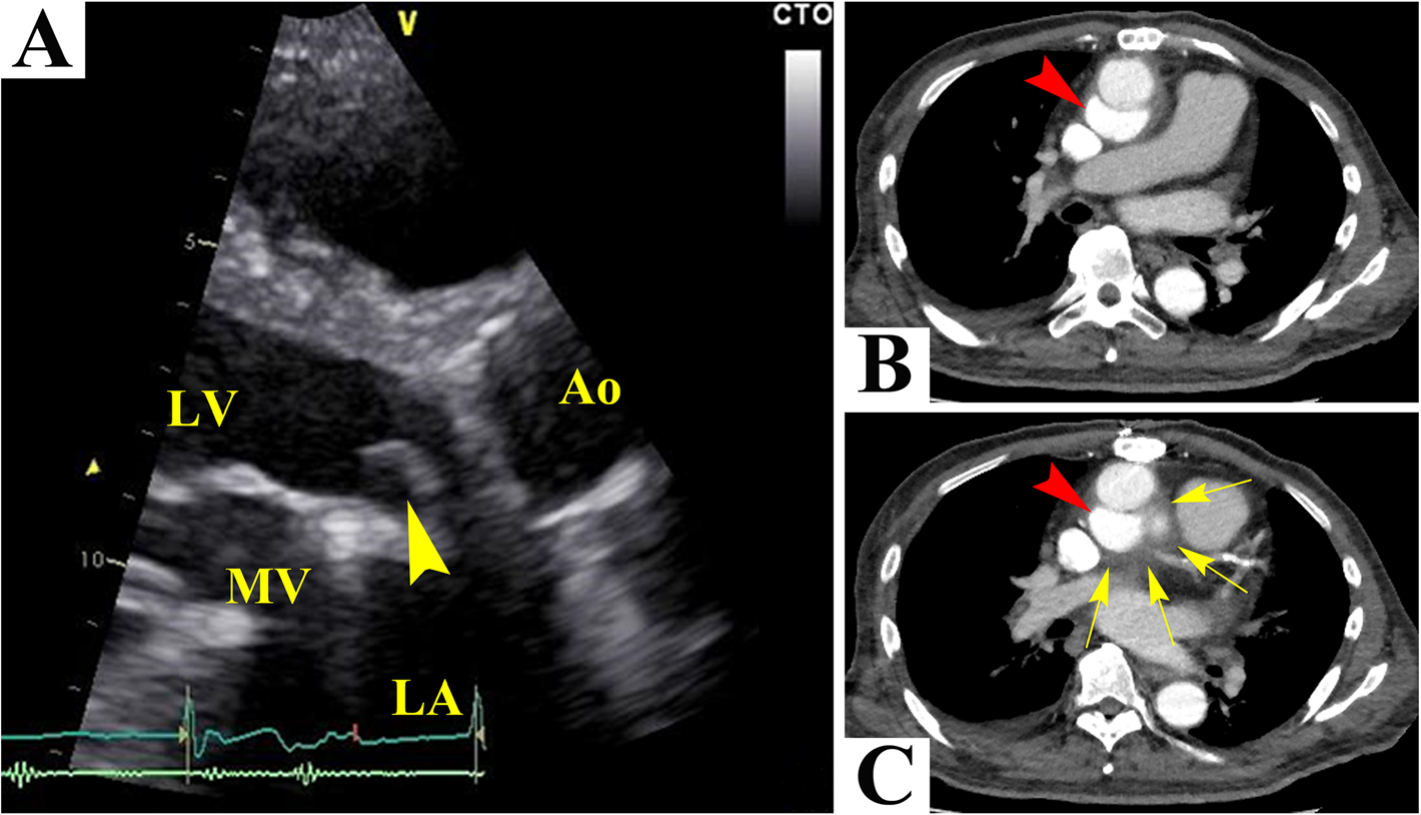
Preoperative examinations. Echocardiography showed infectious endocarditis (IE) in the aortic valve (a, arrowhead). Computed tomography revealed a false aneurysm in the dorsum of the artificial vessel (b, c, arrowheads) and the rise of fat tissue in the mediastinum, indicating mediastinitis (c, arrows)
